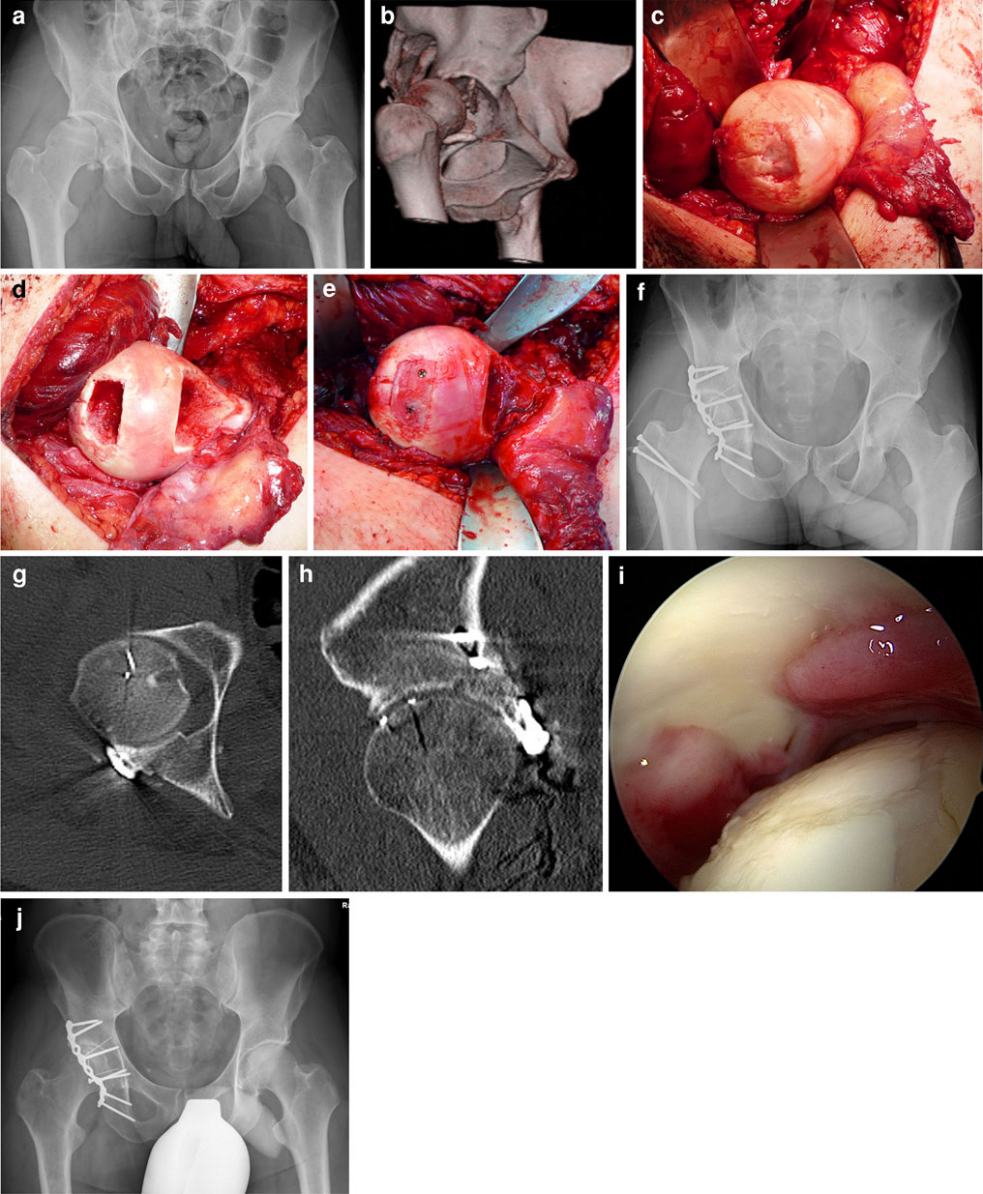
a Anteroposterior radiograph of the pelvis of a 44-year-old patient one week after a car accident demonstrates a partially dislocated femoral head on the *right side*, intraarticular bone fragments, and a comminuted posterior wall acetabular fracture. b Three-dimensional CT reconstruction demonstrates a partially dislocated femoral head on the *right side*, intraarticular bone fragments, and a comminuted posterior wall acetabular fracture. c Intraoperative view of the right femoral head shows the osteochondral defect within the weight-bearing area and the ruptured hip capsule with an intact retinaculum. d View of the femoral head after resection of the damaged femoral area and after the harvesting of an osteochondral shell autograft within the femoral head–neck junction. e Superior view after reconstruction of the articular surface of the femoral head using the graft and fixation with two 1.5 mm screws. f Postoperative anteroposterior radiograph of the pelvis demonstrates anatomic reconstruction of the posterior wall fracture and femoral head damage with osteochondral transplantation. g Axial CT scan demonstrates anatomic reconstruction of the posterior wall fracture and femoral head damage with osteochondral transplantation. h Sagittal CT reconstruction seven months postoperatively shows complete integration of the graft and the two slightly prominent screws in the femoral head, as well as small cyst formation in the dome as a sign of early osteoarthritis. i Arthroscopic view of the acetabular and femoral head cartilages shows moderate chondropathy of the femoral head during hip arthroscopy eight months after trauma from partial removal of the femoral head screws and removal of the trochanter screws. j Anteroposterior radiograph of the pelvis 1 year after surgical hip dislocation
